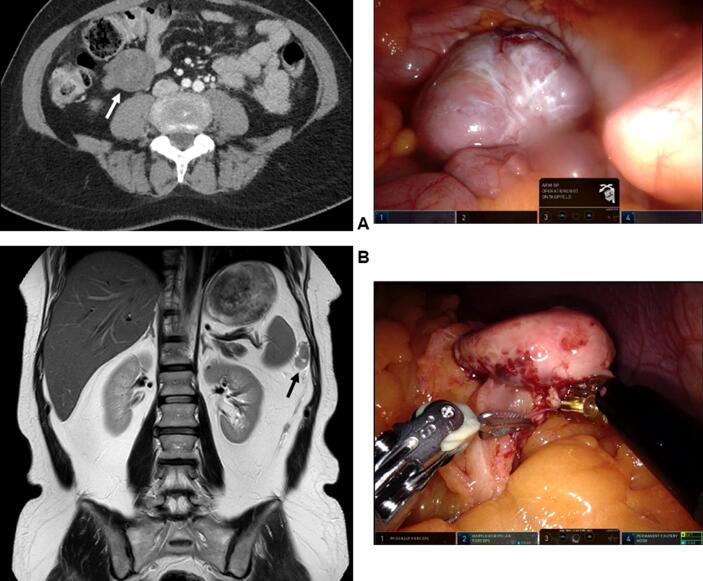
Imaging of recurrent aGCT lesions and correlating image of the robotic surgery. A: Patient 2. The deposit on the mesocolon is shown on CT imaging (left) and during surgery (right). B: Patient 3. A paracolic peritoneal deposit is shown on MRI imaging (left) and during surgery (right).
